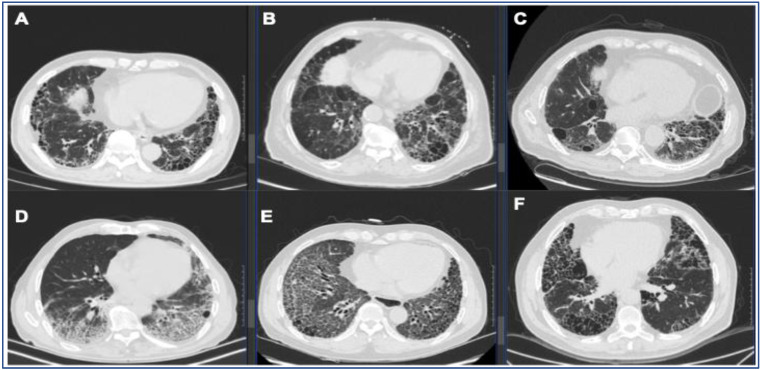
Representative UIP pattern on HRCT in IPF and secondary UIP. (A) IPF, (B) fHP, (C) RA-ILD, (D) pSS-ILD, (E) SSc-ILD, (F) AAV-ILD. Radiologic UIP pattern is defined as basilar, subpleural distribution of reticulation and traction bronchiectasis with honeycombing and without features incompatible with UIP.
